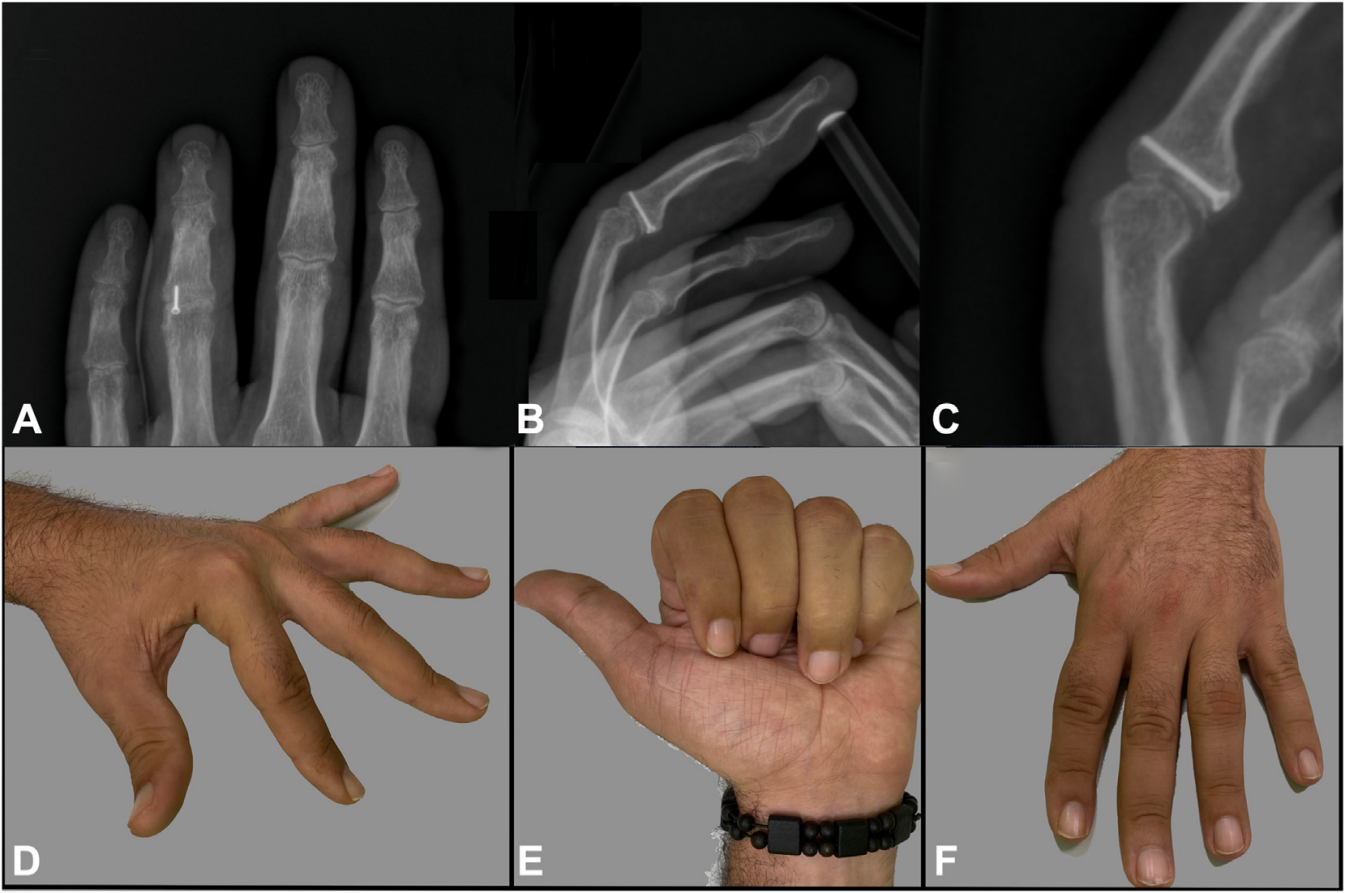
Clinical and radiographing assessment at the 3-month follow-up. A AP view. B LL view. C Detail on articular match and good osteointegration. D Lateral view at the 3-month follow-up. E Palmar view at the 3-month follow-up, corrected overlap. F Dorsal view at the 3-month follow-up, corrected fourth finger angulation.
